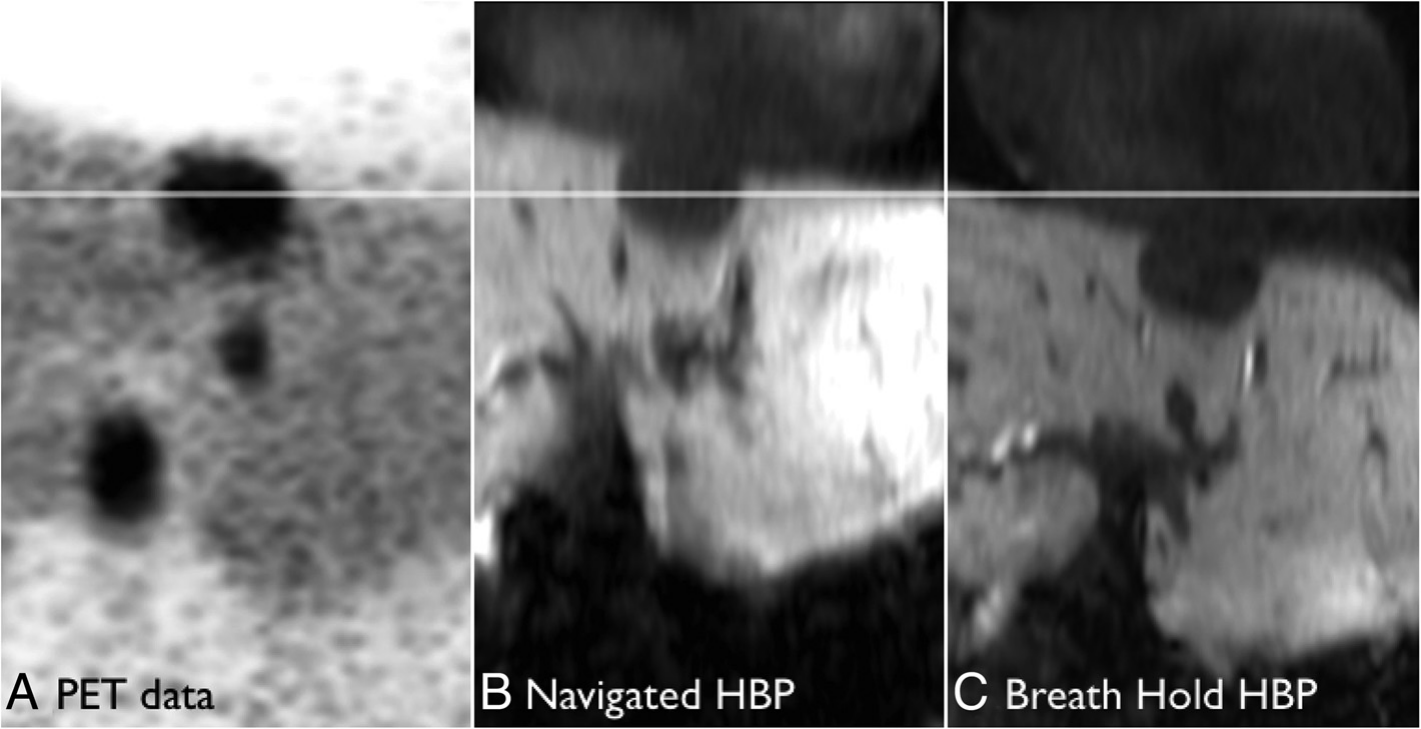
Example case demonstrating accurate localization of MR and PET data using navigated MRI imaging. Respiratory compensated (RC-liver) PET data (a) demonstrates a lesion in segment 2/4A of the liver, which corresponds with a hepatobiliary phase (HBP) hypointense lesion on MR (b and c). Using a navigated acquisition, the lesion is aligned with the PET data (a and b), as compared to a breath-held acquisition where the lesion is displaced inferiorly with respect to the PET acquisition (a and c)
